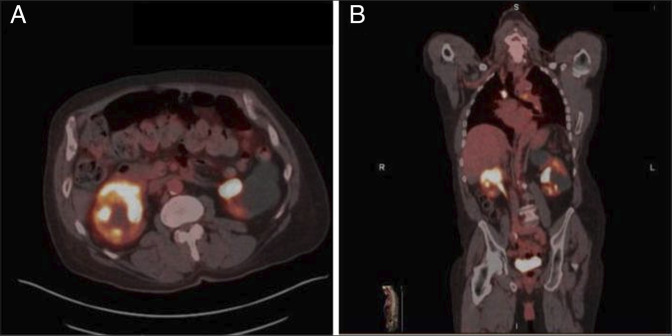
(A) Axial MRI highlighting poorly circumscribed right renal mass with intense FDG uptake. (B) Sagittal MRI demonstrating renal mass and mediastinal lymphadenopathy with FDG uptake. FDG, fluorodeoxyglucose; MRI, magnetic resonance imaging.
